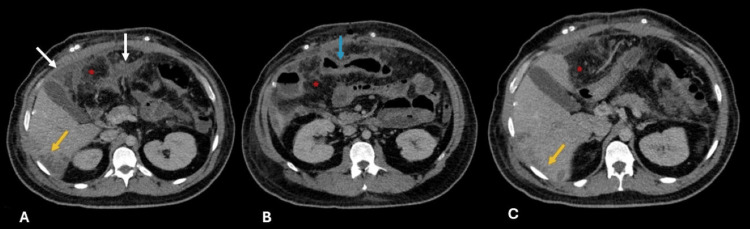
Axial, portal-phase contrast-enhanced abdominal CT A) Confluent intra-abdominal collections (white arrows) and hepatic abscesses in the right lobe (yellow arrows). B) Persistent transverse colon wall thickening with submucosal hypodensity (blue arrow). A-C) Mesenteric fat stranding (red stars) due to inflammatory changes.
